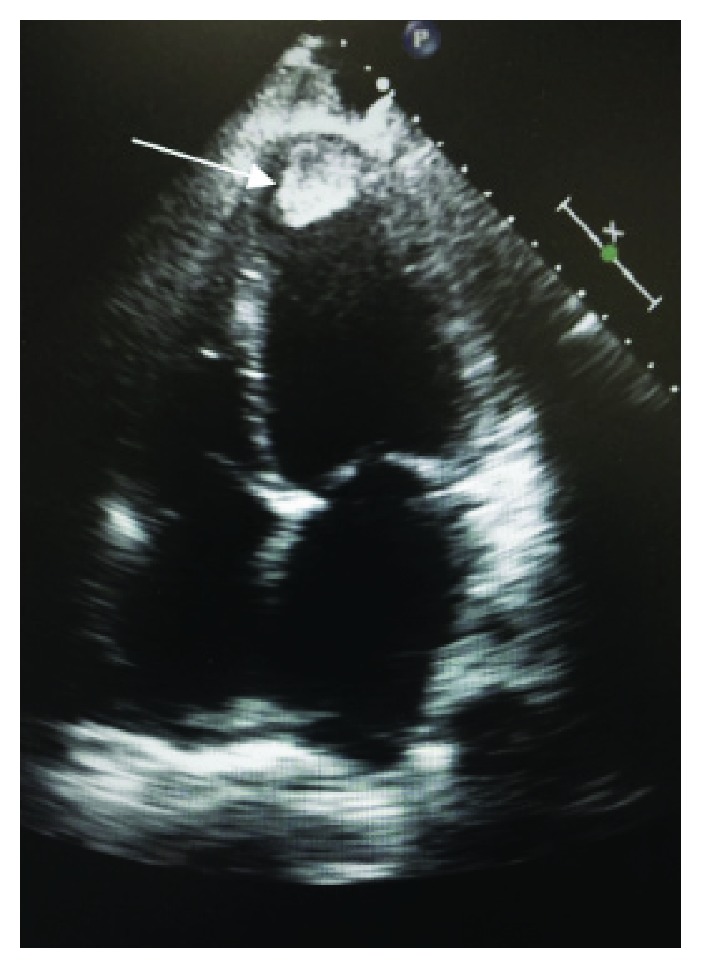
Echocardiogram: an apical four-chamber view showing a 2.2 × 1.4 cm left ventricular thrombus.
